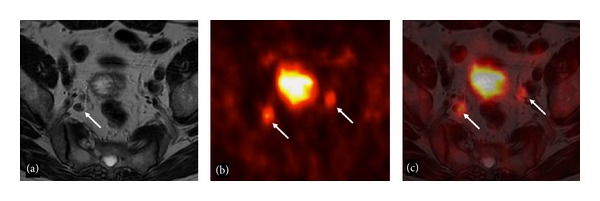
79-year-old man with bilateral hypogastric lymph node metastases. T2-weighted axial image (a) shows a right hypogastric lymphadenopathy (arrow). ^18^F-Choline-PET axial scan (b) demonstrates two areas of focal tracer uptake (arrows). Fused ^18^F-Choline-PET/MRI axial image (c) demonstrates that the left focus of tracer uptake corresponded to a very tiny hypogastric lymphadenopathy (arrows).
